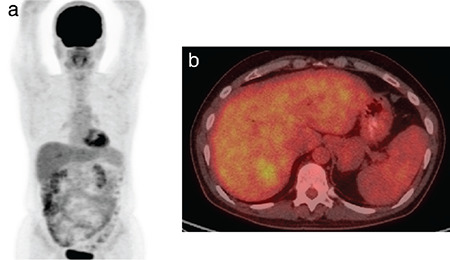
A baseline PET/CT examination of a patient with a solitary liver metastasis from pancreatic carcinoma in the 7^th^ liver segment with SUV_max_ 3.6, which was treated with RFA a week after this study. a) MIP projection, pre-treatment image, b) axial fused pre-treatment image on the level of the 7^th^ liver segment PET/CT: Positron emission tomography/computed tomography, RFA: Radiofrequency ablation, SUV_max_: Maximum standard uptake value, MIP: Maximum intensity projection
